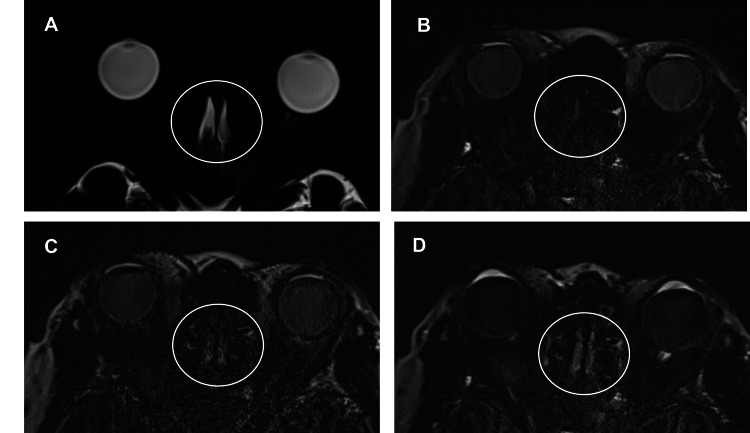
Serial changes in contrast enhancement of the olfactory recess A: The olfactory recess (circle) on magnetic resonance cisternography (MRC). B-D: The olfactory recess (circle) on precontrast (B), postcontrast (C), and 4-h delayed postcontrast (D) HT2-FLAIR imaging. The olfactory recess shows contrast enhancement on postcontrast (C) and 4-h delayed postcontrast (D) heavily T2-weighted (T2W) fluid-attenuated inversion recovery (FLAIR) (HT2-FLAIR) imaging. Contrast enhancement is higher on 4-h delayed postcontrast imaging than on postcontrast imaging.
